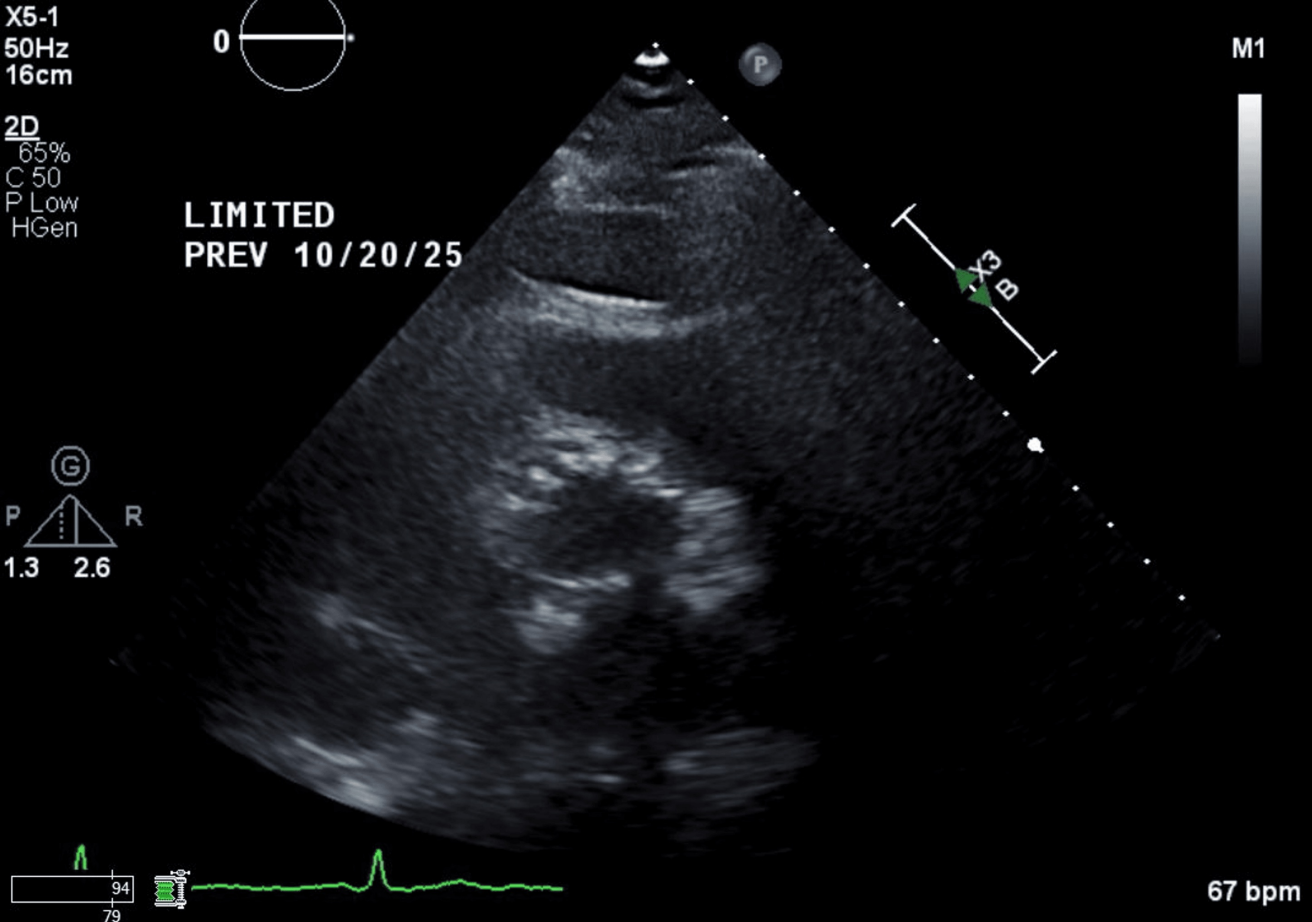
A transthoracic echocardiogram showing the parasternal short-axis view at the level of the aortic valve Post-aortic valve replacement transthoracic echocardiogram demonstrating increased echogenicity around the aortic annulus with a well-seated prosthetic valve
